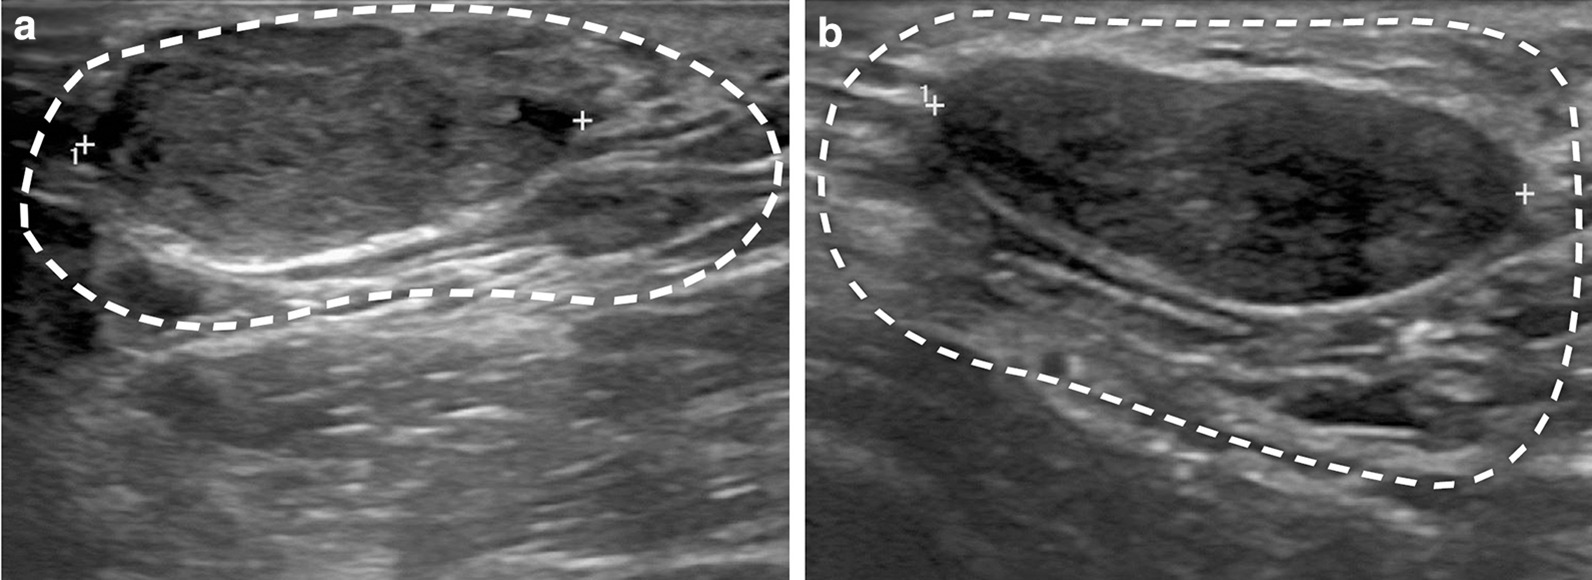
Sonographic findings of a fibroadenoma in an axillary accessory breast. a Accessory mammary gland (AMG) (dashed outline) surrounding an oval-shaped fibroadenoma. In the image, the top of the fibroadenoma is close to the skin, and at the bottom of the fibroadenoma, the AMG is visible surrounding the fibroadenoma. b AMG (dashed outline) surrounding an elliptical-shaped fibroadenoma. The AMG surrounds the fibroadenoma
